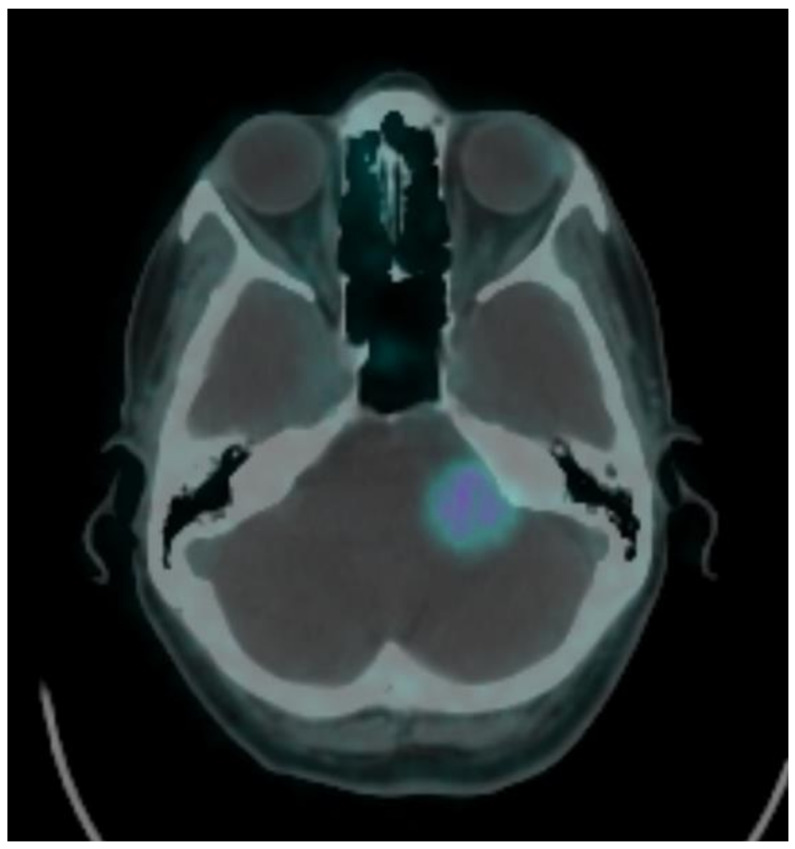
^68^Ga-DOTATOC PET/CT follow up at 6 months showing SUV intensity and reduction of the lesion from 7.1 to 4.1 cm.
